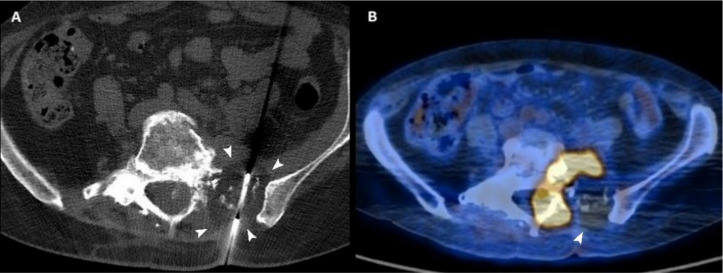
(A) Axial CT scan of a 54-year-old woman with a sacral metastasis from endometrial sarcoma treated with cryoablation for palliative intent. The ice ball is visible as a hypodense circle surrounding the tip of the needle (arrowheads). (B) 18F-FDG PET/CT scan performed 3 months after the procedure demonstrates the absence of pathologic radiotracer uptake in the ablated area (arrowhead).
